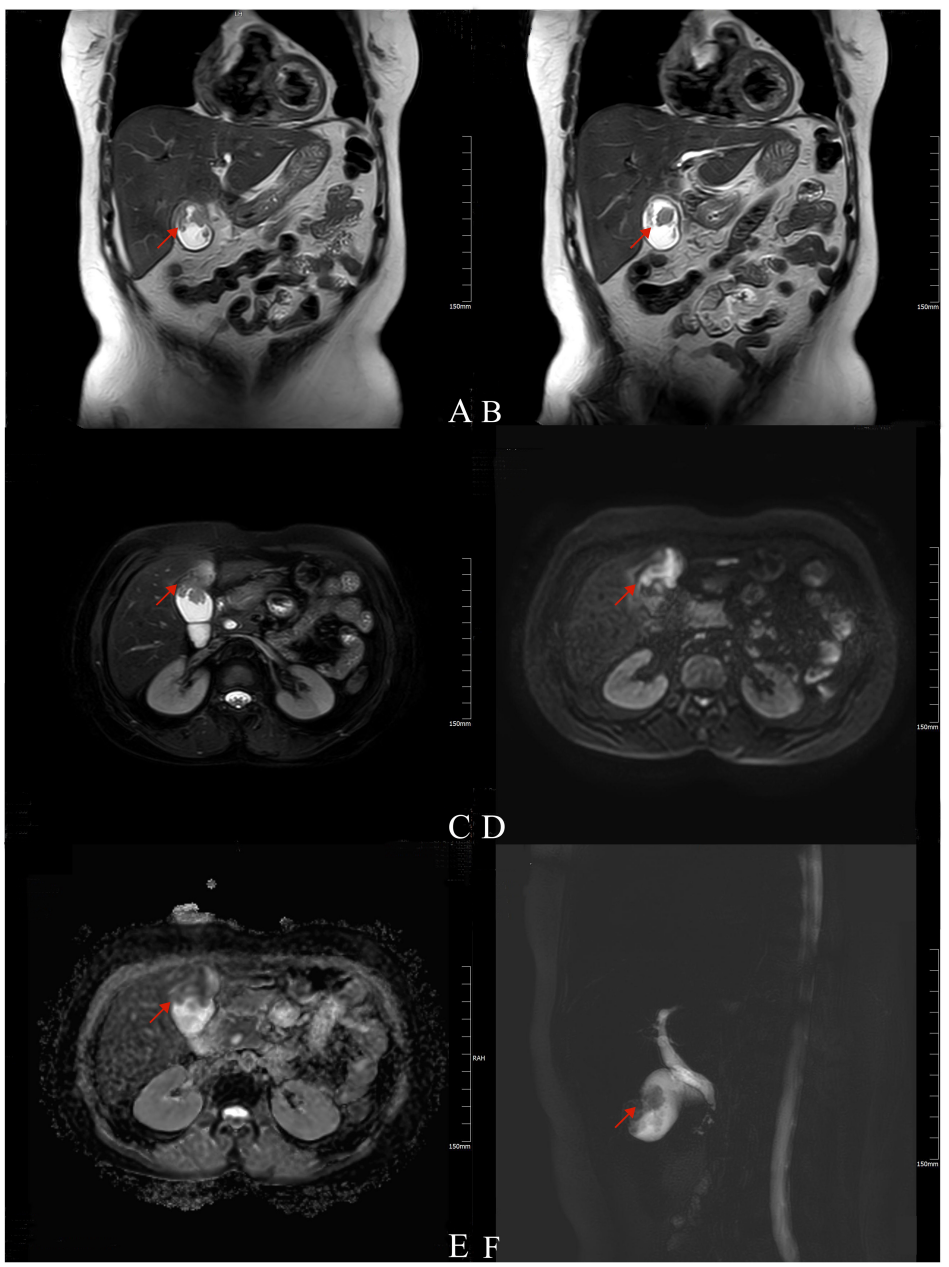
Magnetic resonance imaging (MRI) findings of the patient. T2-weighted (A,B, coronal; C, axial) imaging showed low signal intensity as an irregular filling defect in the gallbladder fundus and long signal in segment IV of the near liver (arrow). DWI (D) showed a hyperintense signal, and ADC map (E) showed reduced diffusion (arrow). MRCP (F) showed gallbladder enlargement with local filling defect (arrow).
